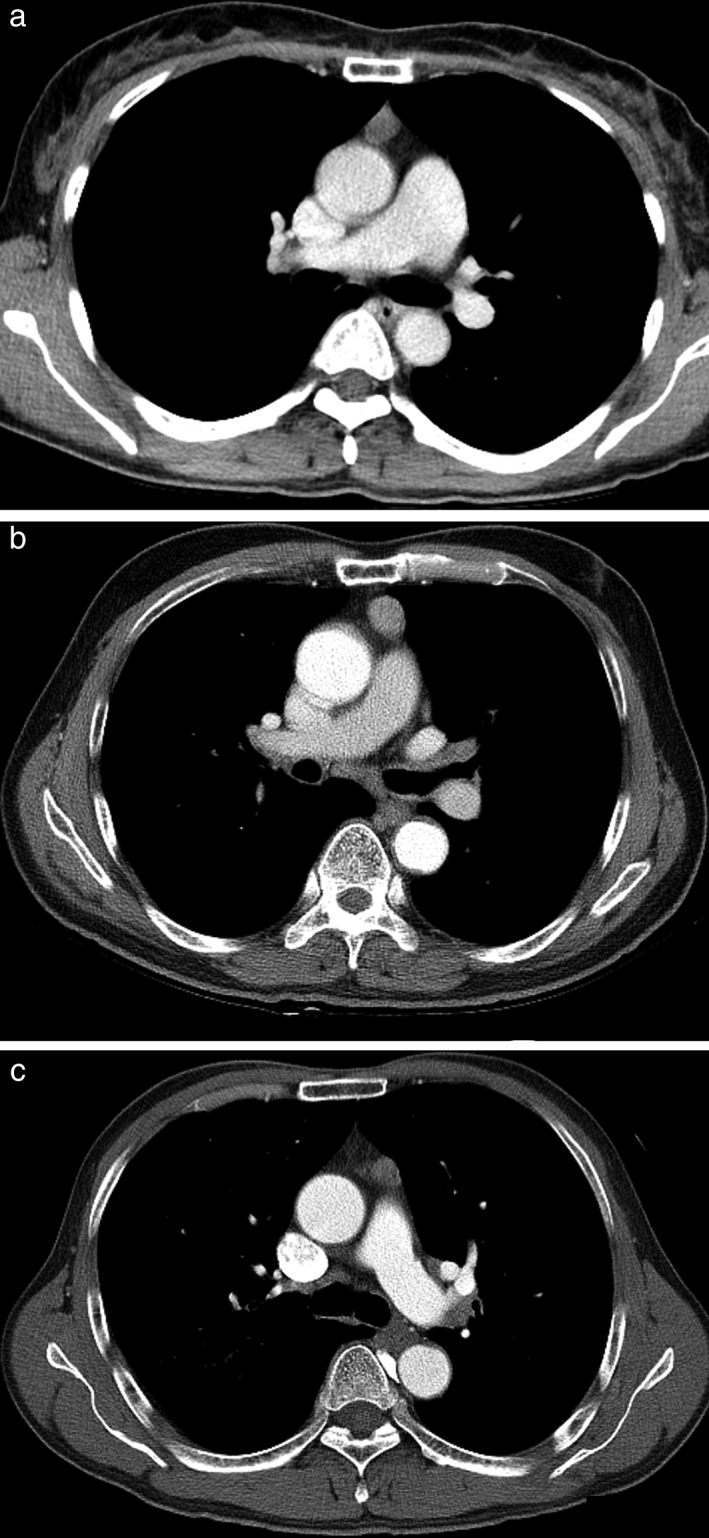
Three CT scans from three different patients showing the limitation of conventional CT in differentiating three pathologies. All scans showed 1 to 2 cm sized smooth‐contoured, median‐located, and soft tissue‐attenuated (>20 HU) anterior mediastinal lesions. The pathology indicated these to be thymic cyst (a), thymoma (b), and thymic carcinoma (c), respectively.
